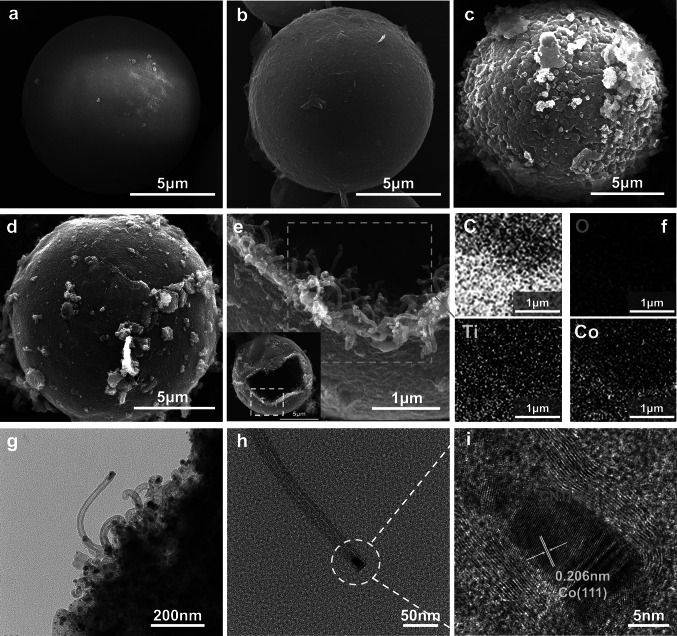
SEM images of a PS, b PS@MXene, c PS@MXene@ZIF67, and d HMCCo-2. e SEM images of an open HMCCo-2 spherical shell and the internal structure of the spherical shell. f Elemental mappings of HMCCo-2. g TEM image of the edge of the sample fragments obtained after ultrasonication, including carbon nanotubes grown in situ. h TEM image of a single carbon nanotube separated by ultrasound. i HRTEM image corresponding to the white circle in (h)
